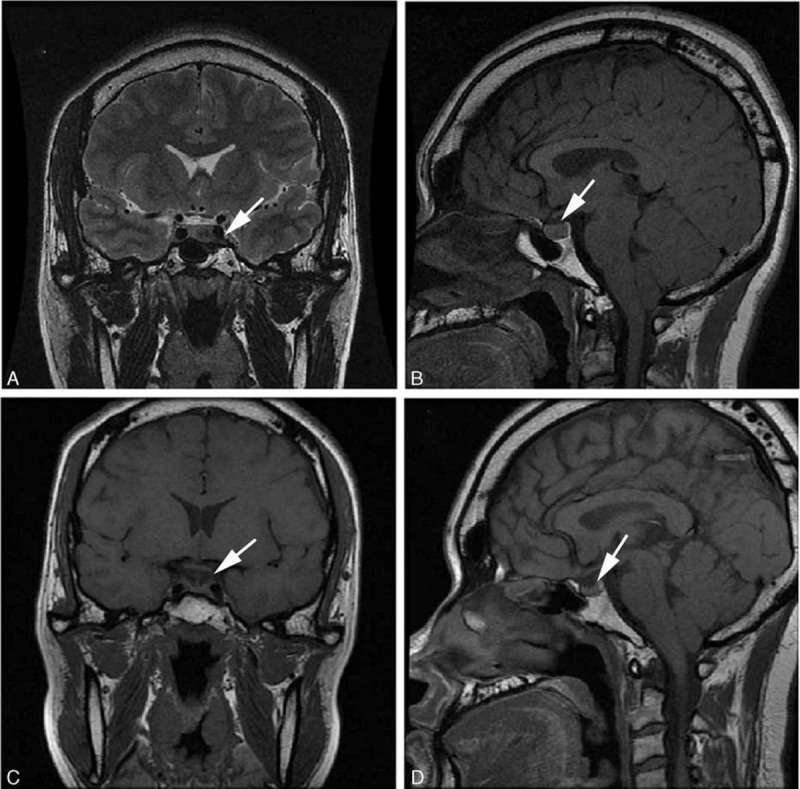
Head MRI. (A, B) A preoperative postcontrast coronal image shows a 9 × 7 × 6 mm slightly low-density shadow with an unclear border (white arrow). (C, D) After the operation, MRI showed no obvious abnormal signal in the lesion (white arrow).
